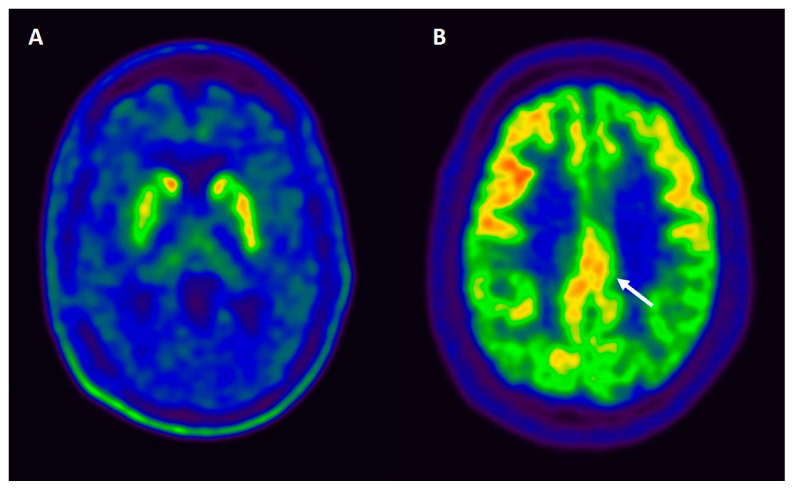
(A) Axial ^18^F-Dopa PET image of a patient with dementia with Lewy bodies. The image shows a moderate decrease of bilateral striatal activity (dopaminergic denervation), more pronounced in the right putamen. (B) FDG-PET findings in a patient with dementia with Lewy bodies. Axial FDG-PET image demonstrates the cingulate island sign (indicated with an arrow), which reflects preservation of posterior cingulate metabolism relative to cuneus and precuneus.
